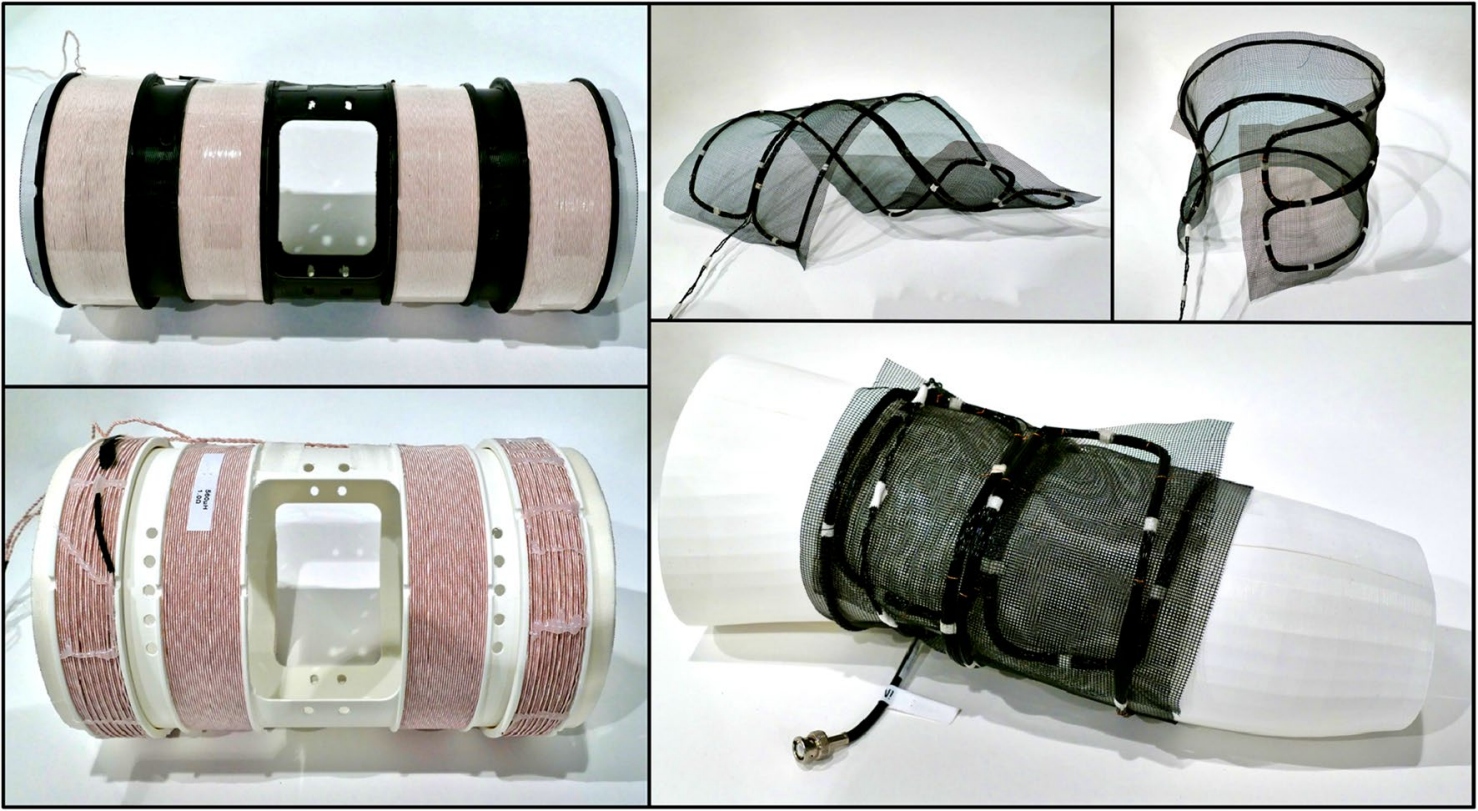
Two different approaches for receive coils are demonstrated: left: the rigid-body receive coils with different diameters (*d*_black_ = 11 cm, *d*_white_ = 18 cm) provide four coils each to be used as gradiometer. Both provide a dedicated X-ray window. Right: a flexible receive coil consisting of two racetrack coils assembled on a flexible material.
